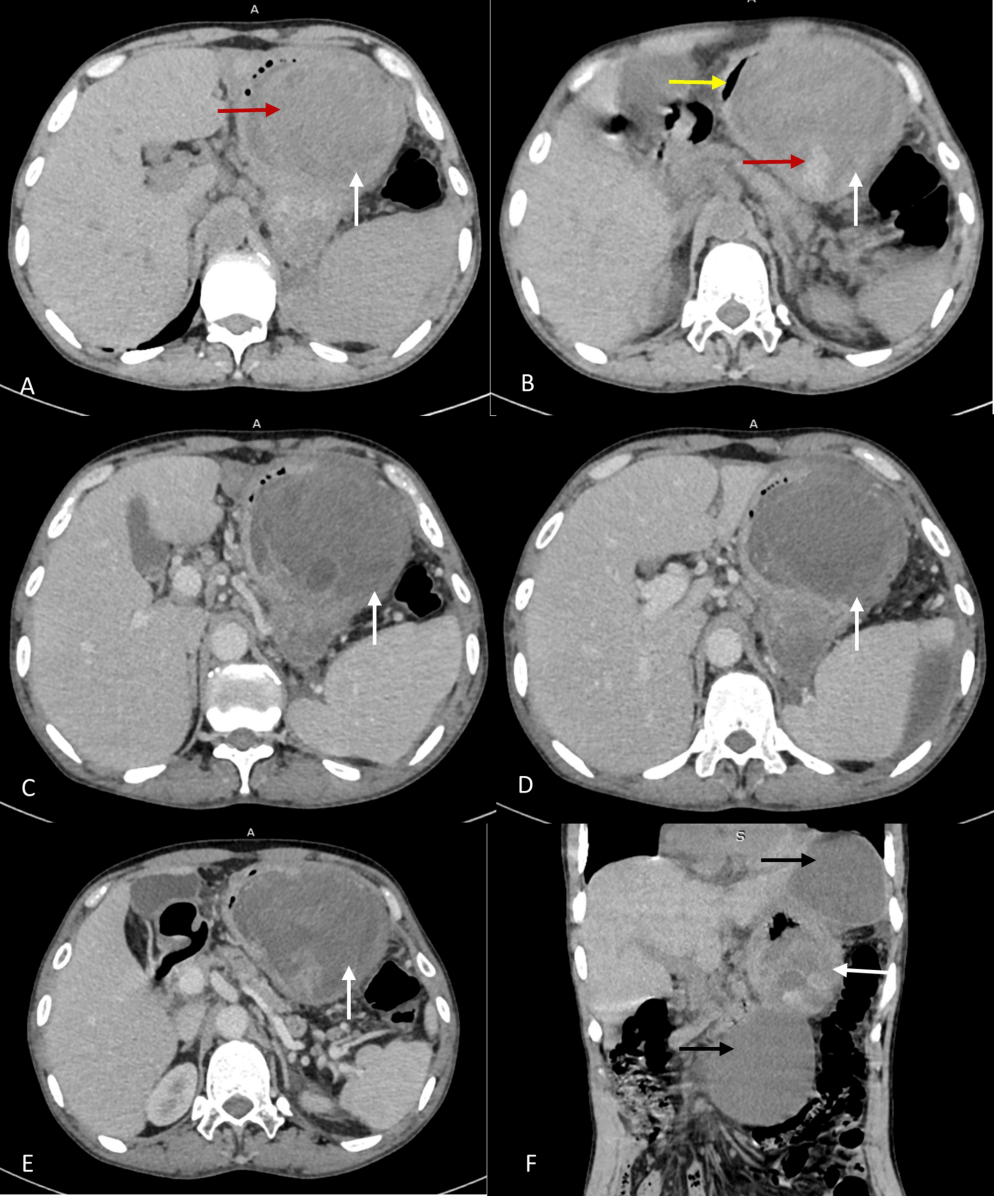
NCCT and CECT of abdomen and pelvis. (A) and (B): NCCT axial views; (C)-(E): CECT axial views images show well-defined hypodense lesion (pseudocyst-white arrows) with hyperdense and heterogeneous areas (hemorrhage-red arrows) in the lesser sac causing significant mass effect on stomach (yellow arrow). (F) NCCT coronal view: image shows three well-defined hypodense lesions in the lesser sac and epigastric region (uncomplicated pseudocysts-black arrows and hemorrhagic pseudocyst-white arrow). The lesion in the lesser sac with hyperdense and heterogeneous areas. NCCT: non-contrast computed tomography, CECT: contrast-enhanced computed tomography.
